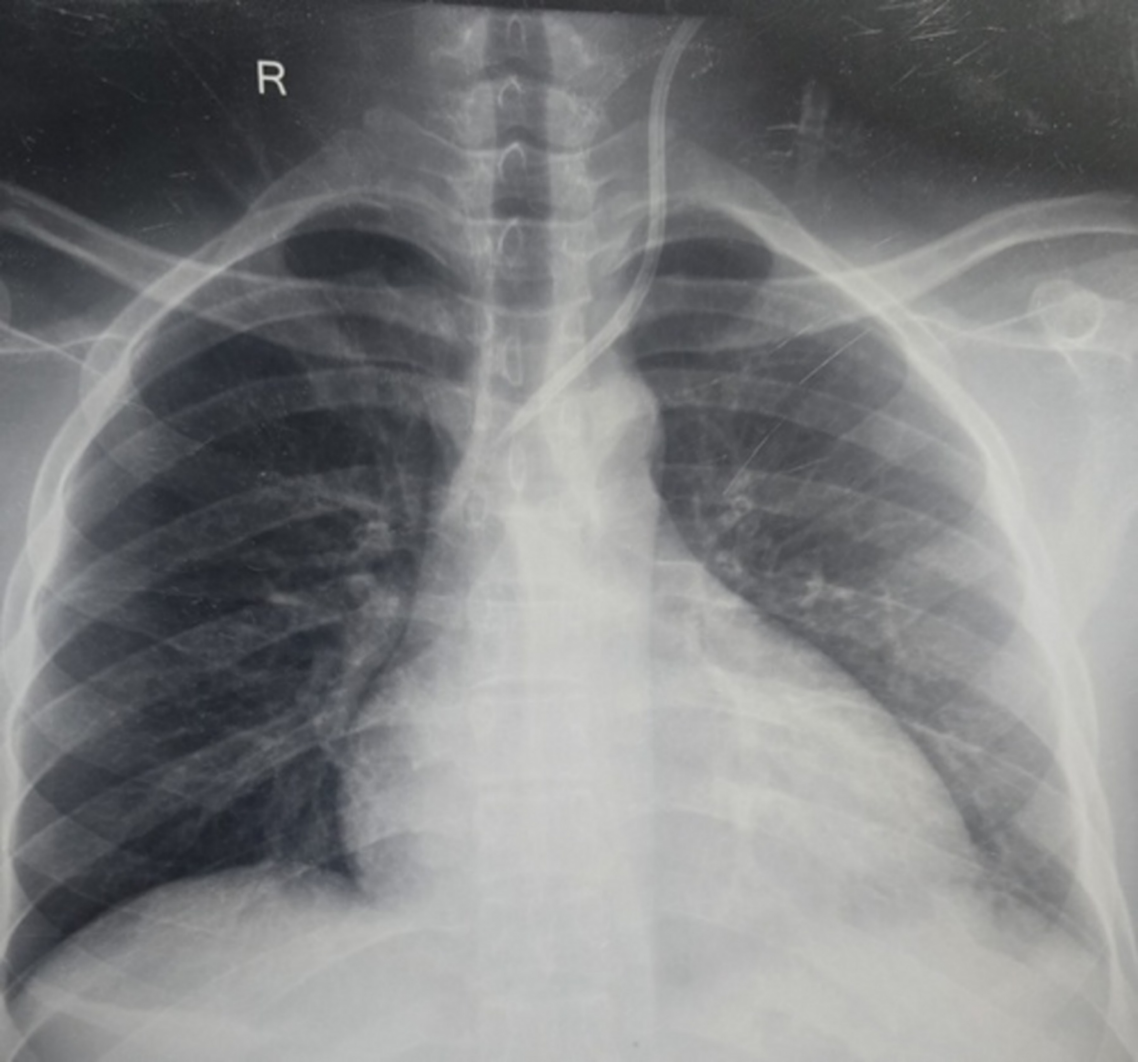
X-ray showing normal course of left IJV catheter. IJV: internal jugular vein
